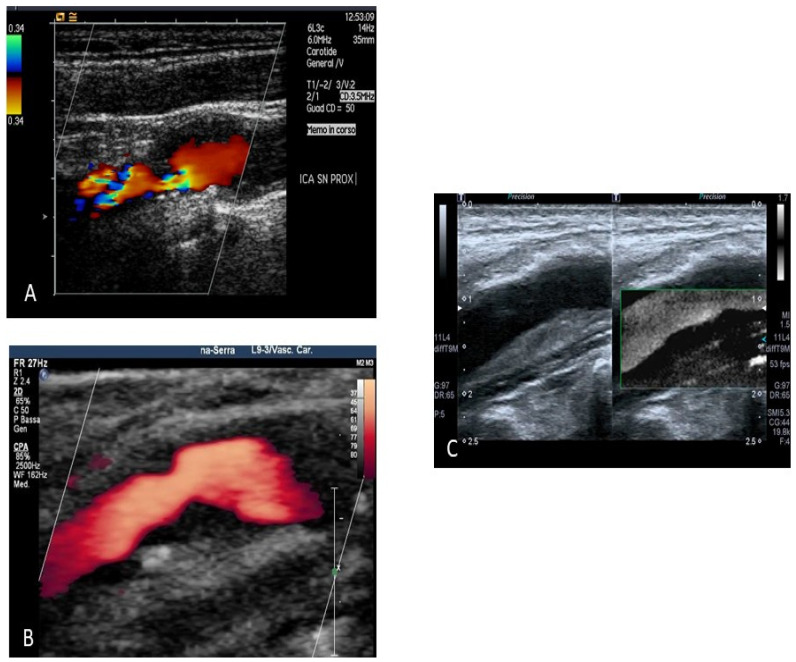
Duplex ultrasound (DUS; (A,B)) and contrast-enhanced ultrasound (CEUS; (C)) of vulnerable carotid plaque. (A) Colour Doppler image of the proximal left internal carotid artery showing an eccentric, predominantly hypoechoic (echolucent) bulb plaque with luminal narrowing and a turbulent, aliased flow signal indicating a haemodynamically significant stenosis. Low plaque echogenicity (low grayscale median) is the ultrasonographic correlate of a soft, lipid-rich necrotic core; a juxtaluminal hypoechoic (“black”) area lacking an overlying echogenic cap is, in addition, associated with intraplaque haemorrhage and an increased risk of cap rupture. (B) Colour Doppler image demonstrating an irregular luminal contour with a focal recess into which colour flow extends, consistent with plaque ulceration and surface (fibrous-cap) disruption. (C) Dual-screen CEUS examination: microbubble contrast sharpens delineation of the luminal surface and can demonstrate intraplaque enhancement; intraplaque neovascularization on CEUS is a recognised marker of plaque inflammation and instability and correlates with intraplaque haemorrhage.
